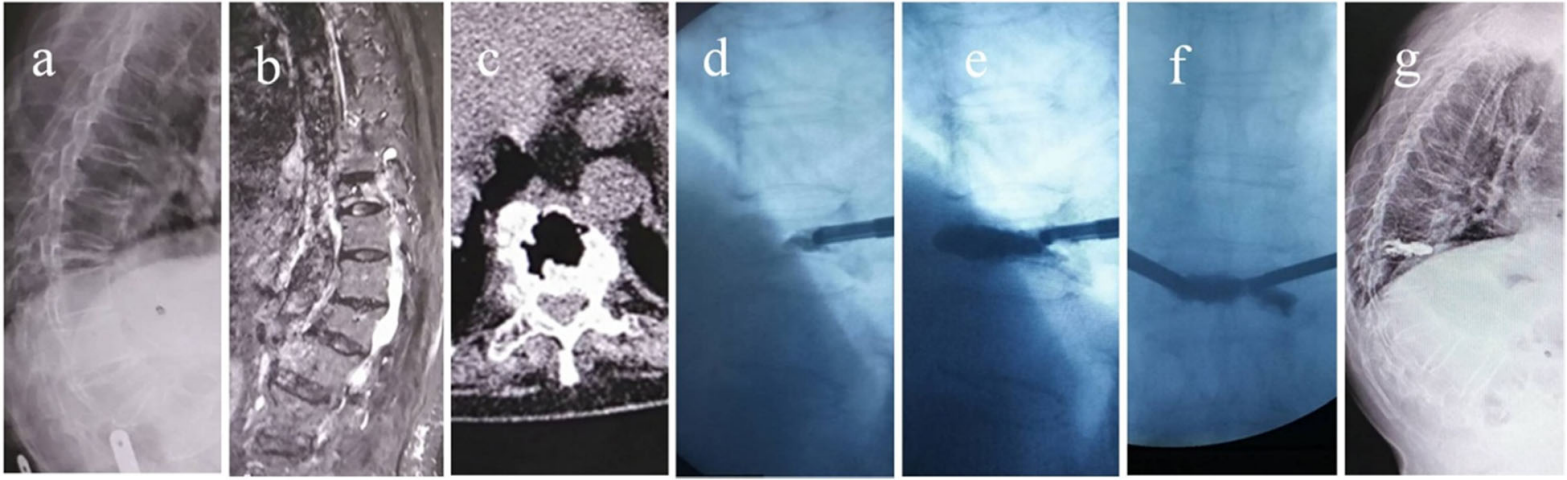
Preoperative, intraoperative and postoperative imaging findings of Kümmell’s disease. a Sagittal X-ray image showing the extreme compression and thinning of the diseased vertebrae. b Sagittal STIR image showing a band-like low intensity signal. c Coronal computed tomography image showing Th8 intravertebral fluid and air-densities. d The bone cement injection in the beginning showed that the bone cement penetrates forward. e Sagittal C-arm image showed the favorable penetration of bone cement. f Coronal C-arm image showed the favorable penetration of bone cement. g Sagittal X-ray image postoperatively showed the favorable penetration of bone cement
